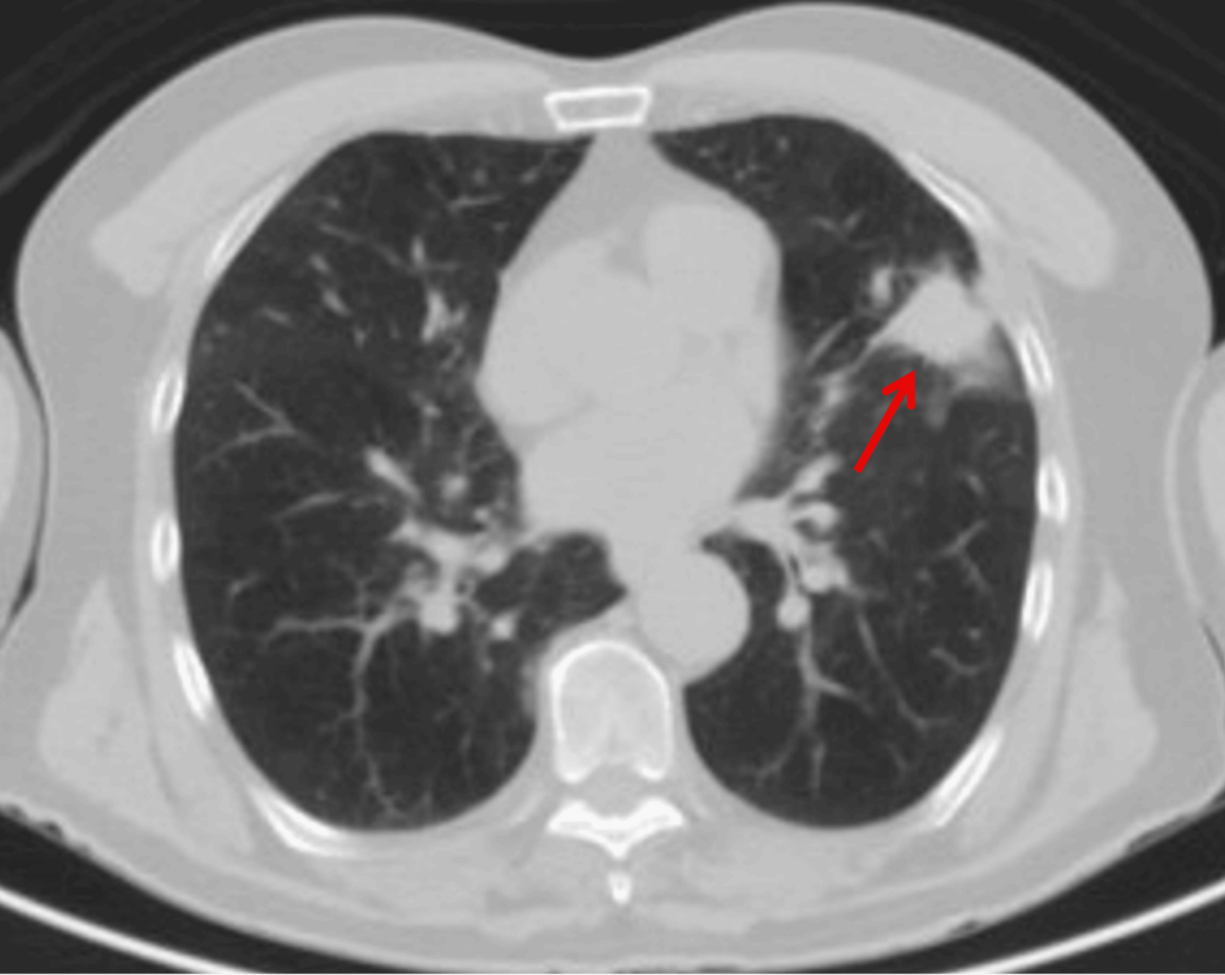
Chest CT showing a spiculated pulmonary mass in the left upper lobe Axial chest computed tomography (CT) scan demonstrates a spiculated mass (red arrow) in the lingular segment of the left upper lobe, measuring 3.0 × 2.4 × 1.7 cm. Surrounding moderate emphysematous changes are also noted.
